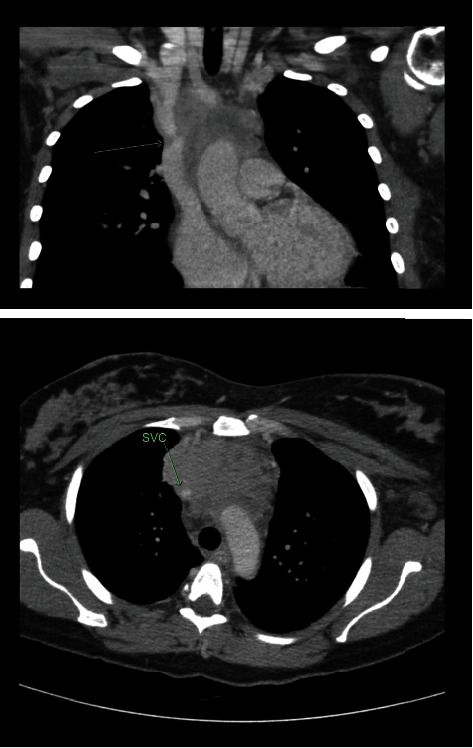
CT scan on presentation showing a mediastinal mass and concomitant obstruction of the SVC to 1 mm in diameter (green arrow).
